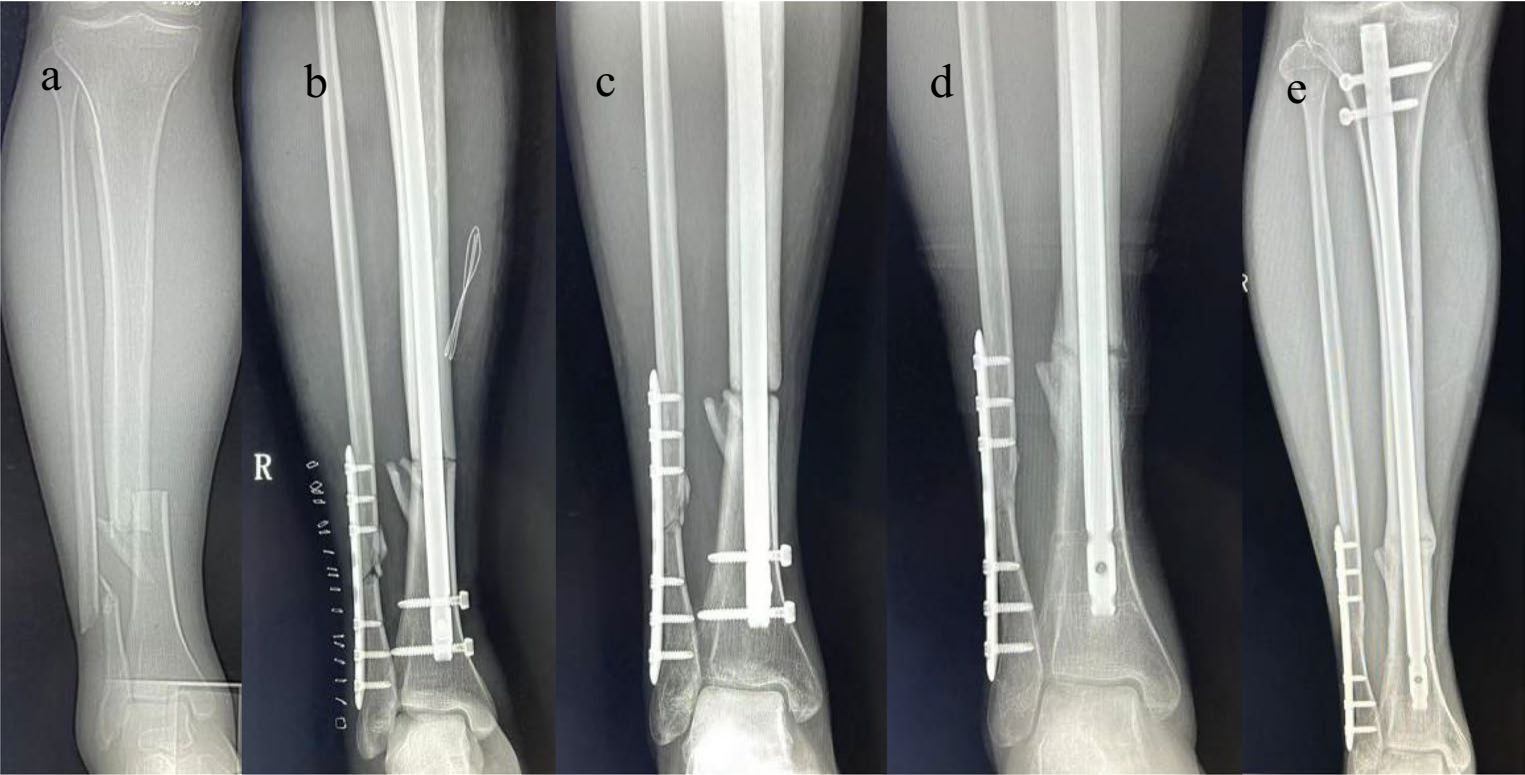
Radiographs showing delayed healing after 1.5 years of follow-up after intramedullary nailing of a distal tibia fracture. (a) Preoperative radiographs with a TFI ratio of 1.45; (b) postoperative reexamination of radiographs showed poor alignment, and the anteroposterior radiographs were inverted at an angle of 4°; (c) 5 months after surgery, bone resorption occurred at the fracture end, an obvious fracture line was observed, and delayed healing occurred; (d) 5 months after the distal locking device was removed, reexamination of the radiographs revealed blurred fracture lines and callus junction fractures; (e) 1 and a half years after surgery, the fracture line was barely visible, a callus junction fracture occurred, and fracture healing occurred
